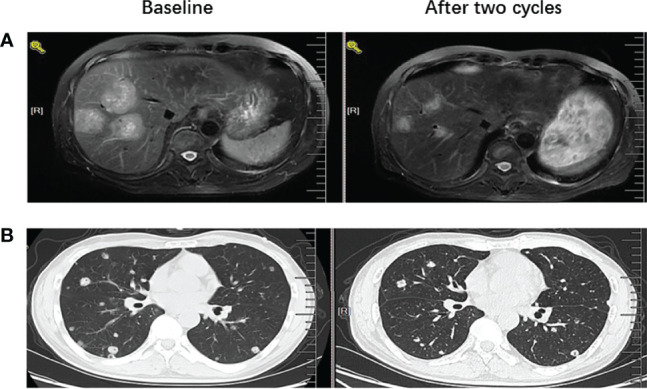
MRI or CT images before and after treatment to show PR and SD results (A) left image showed baseline MRI images for one patient with liver metastases before the treatment with fruquintinib plus pd-1 inhibitor and right image showed the tumor reduction more than 50% after two cycles of treatment. (B) left image showed baseline CT images for one patient with lung metastases before the treatment with fruquintinib plus pd-1 inhibitor and right image showed the tumor remain stable after two cycles of treatment.
